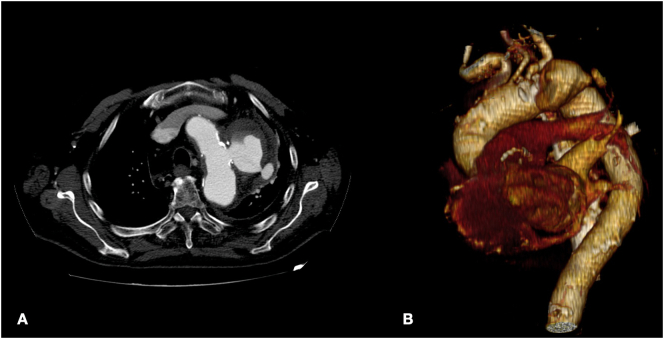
Preoperative CT Angiography (A) and 3-dimensional volume rendering (B) showing aneurism of aortic isthmus.
